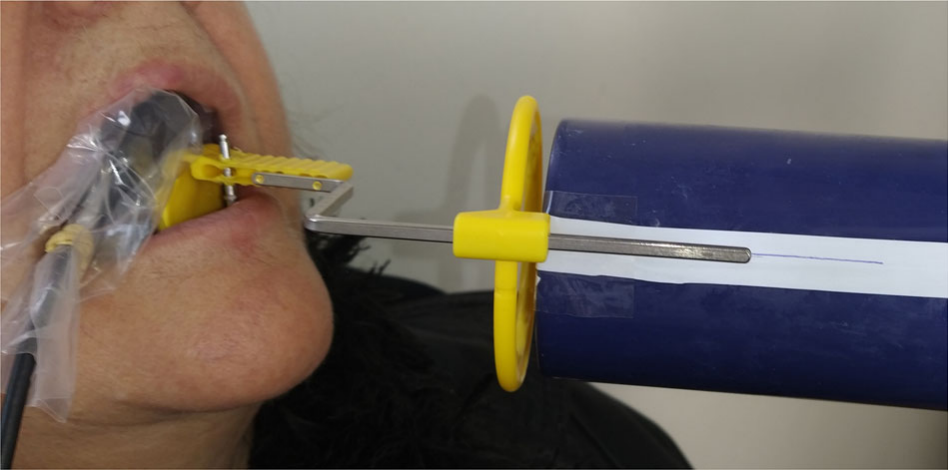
Parallel periapical X-ray image using the sensor holder. The X-ray beam was aligned perpendicular to the sensor using the metal arm and circular guide.
